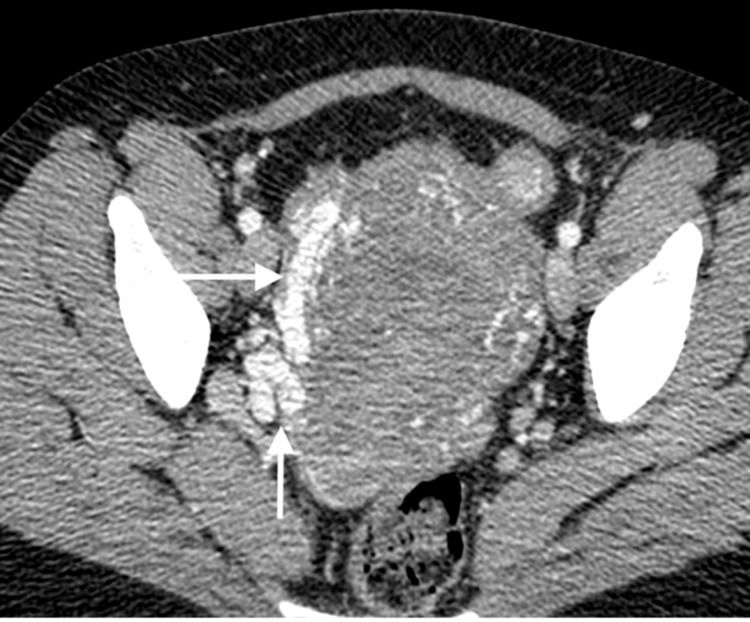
Axial, arterial phase post-contrast CT, through the pelvis, showing engorged, tortuous, right uterine artery (vertical arrow) and early filling of the right ovarian vein (horizontal arrow).
